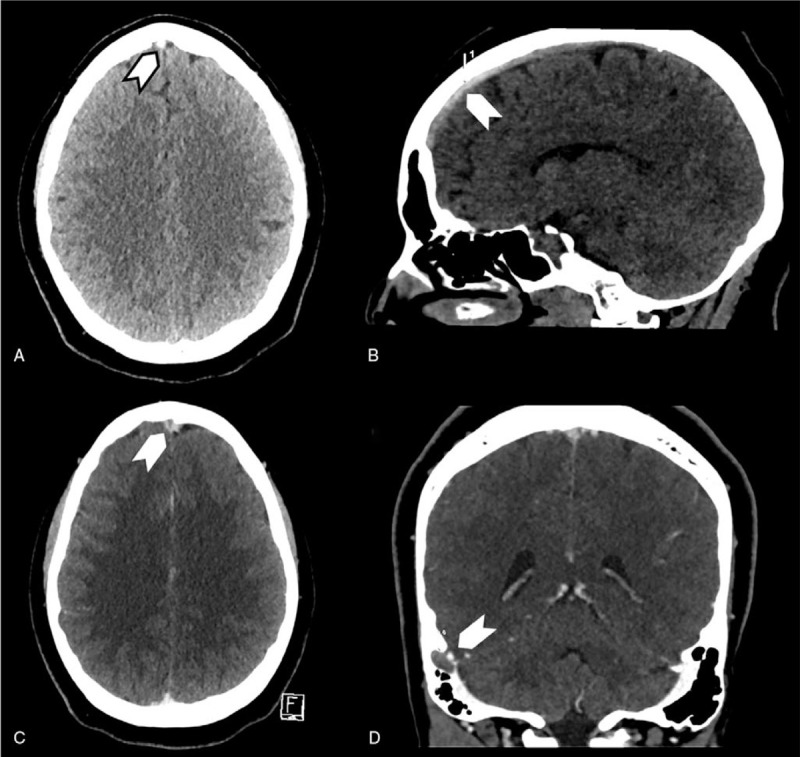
Patient (case 2) cerebral CT scan showing cerebral venous thrombosis. A (axial view) and B (sagittal view) showing spontaneous density of the anterior part of the superior sagittal sinus (arrows), C (axial view) and D (sagittal view) with contrast showing filling defect in the superior sagittal sinus with classical delta sign (C) and in the right sigmoid sinus (D) (arrows).
